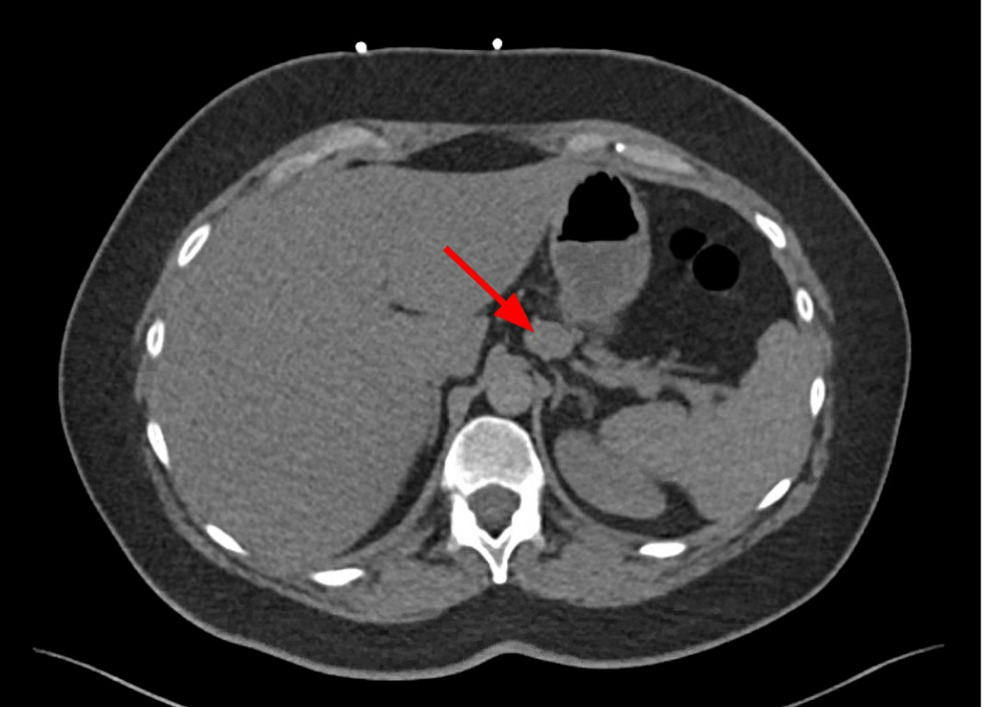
CTA showing a 2.2 cm exophytic lesion in the pancreas (red arrow); MRI recommended by the radiologist for further characterization of the lesion. CTA, computed tomography angiography
